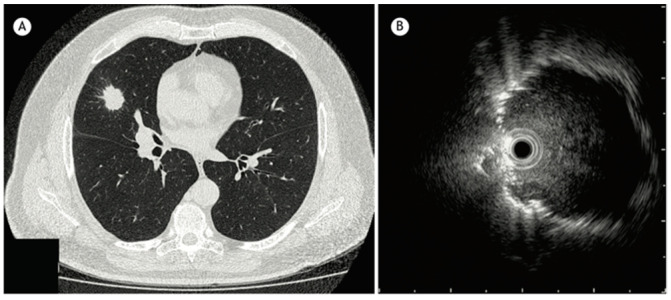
(A) CT chest showing a 2.5 cm nodule in the middle lobe. (B) Radial EBUS image reproduced from Jacomelli et al., 2015 [13] (Shared under the terms of the Creative Commons Attribution License, https://creativecommons.org/licenses/by/4.0/).
